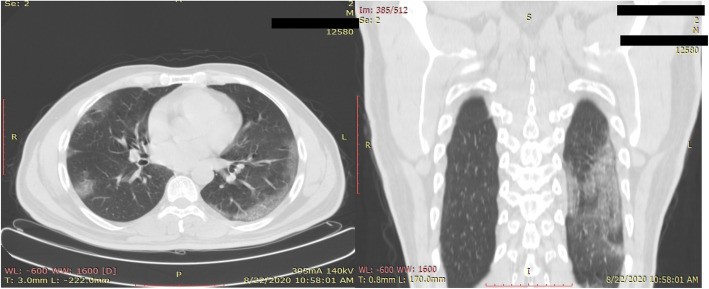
A 36-year-old male patient with positive PCR test. Chest CT shows bilateral ground glass opacities in a peripheral/subpleural distribution with lung involvement of 5–25%, i.e., CT severity score of 2
